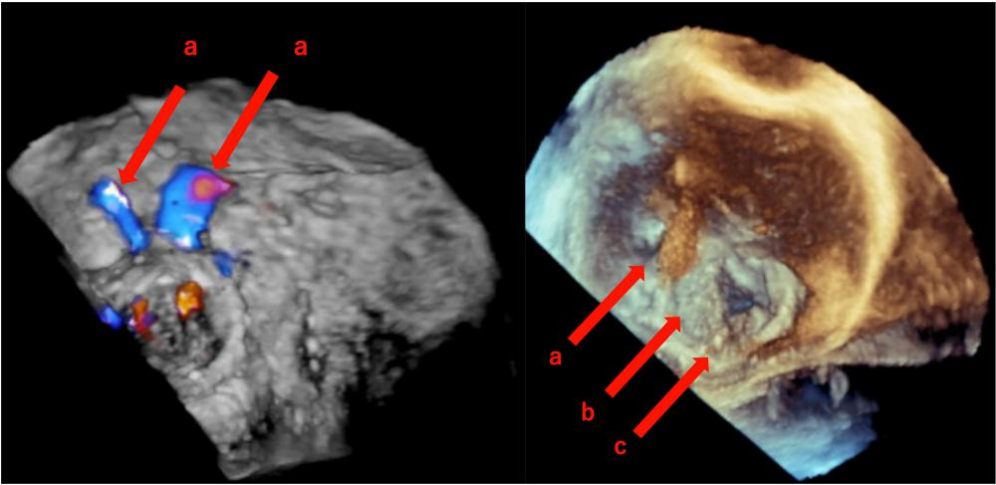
3D transoesophageal echocardiography. (*A*) Para-valvular leakage. (*B*) Closed stuck valve. (*C*) Thrombus.
